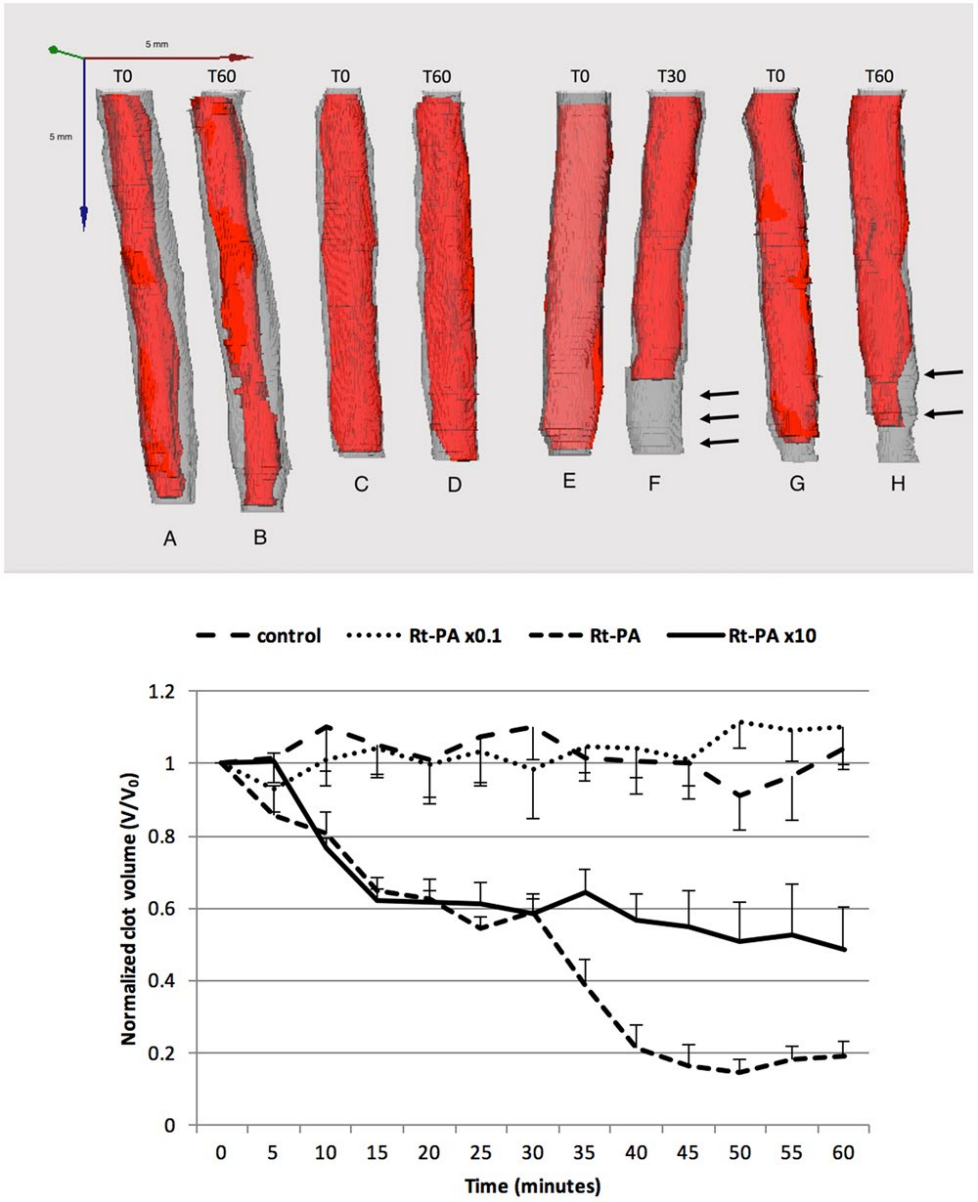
Thrombolytic efficacy of *in vitro* after Rt-PA delivery in the stenosis model. Blood clots were treated with a range of Rt-PA alone in a range of concentrations (0, 0.3, 3, 30 µg/ml). The blood clot volume was assessed every 5 min for 60 min using high frequency ultrasound imaging. 3D reconstruction of clots was performed at T0 and T60 (Top), except for the Rt-PA condition (T30) as the clot was almost lysed at T60. A, B Rt-PA x0,1; C-D: control; E-F: Rt-PA; G-H: Rt-PAx10 (Arrows indicating the lysis of the clot). Video 1 is showing the clot volume at T0 and T30 in the Rt-PA condition. Data expressed as mean ± SEM were calculated from four independent experiments (Bottom).
